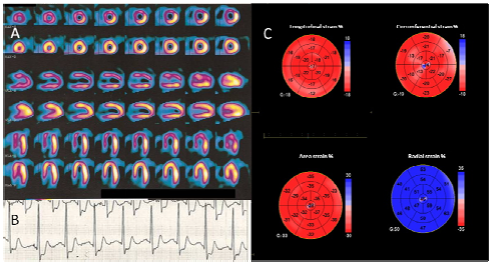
Perfusional Imaging. A: Single Photon Emission Computed Tomography showing severe myocardial perfusion abnormalities of the interventricular septum and the apex. B: Representative lead (V5) with ST segment depression during exercise stress test. C: Three dimensional global and regional longitudinal, circumferential, area and radial strain represented by a bull-eye graph showing no regional deformation abnormalities.
